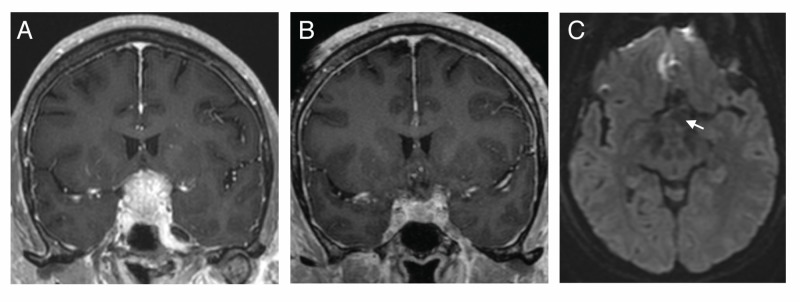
Characteristic pre- and postoperative magnetic resonance imaging (MRI) A) Preoperative coronal T1 post-gadolinium MRI scan revealing homogeneously enhancing mass encasing bilateral internal carotid arteries and causing optic nerve/chiasm elevation. B) Postoperative coronal T1 post-gadolinium MRI scan revealing near-total resection. C) Postoperative axial diffusion-weighted sequence revealing no definitive reduced diffusion along the optic nerves or chiasm (denoted by the white arrow).
